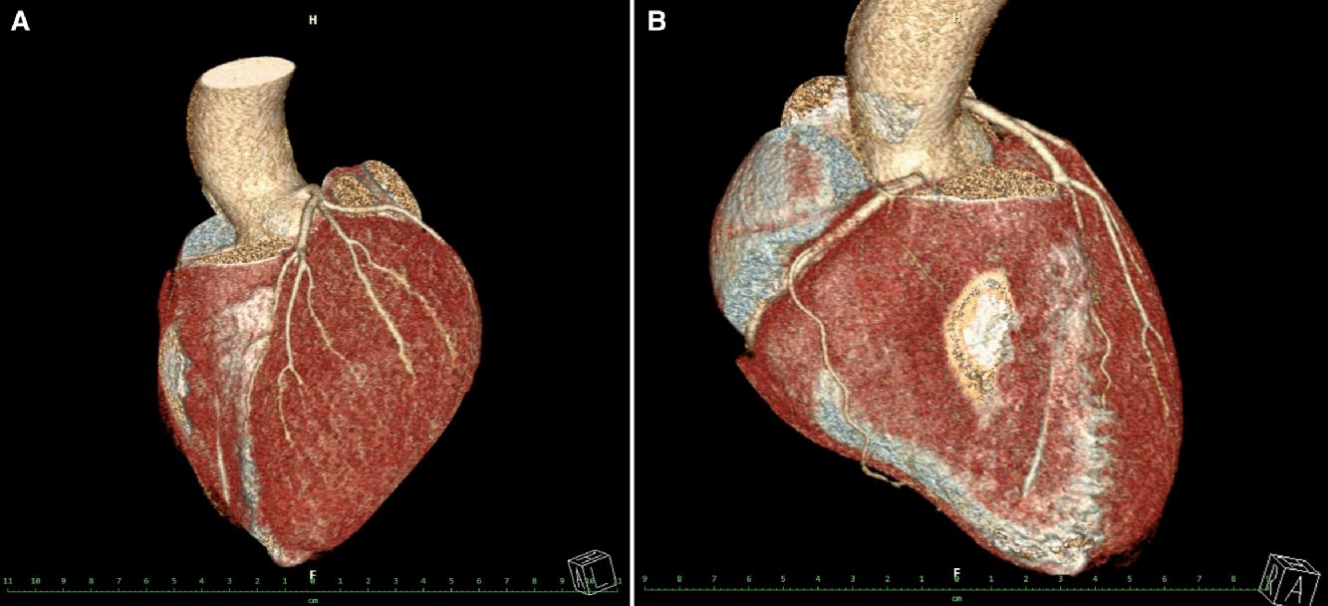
3D reconstruction of coronary computed tomography angiography. (*A*) Displaying left main coronary vessel and its branching (arrow) with no obstruction or other pathology. The dimensions and structure of other heart chambers are within range. Also aortic root is not dilated. (*B*) Showing the right coronary artery territory without obvious pathology.
